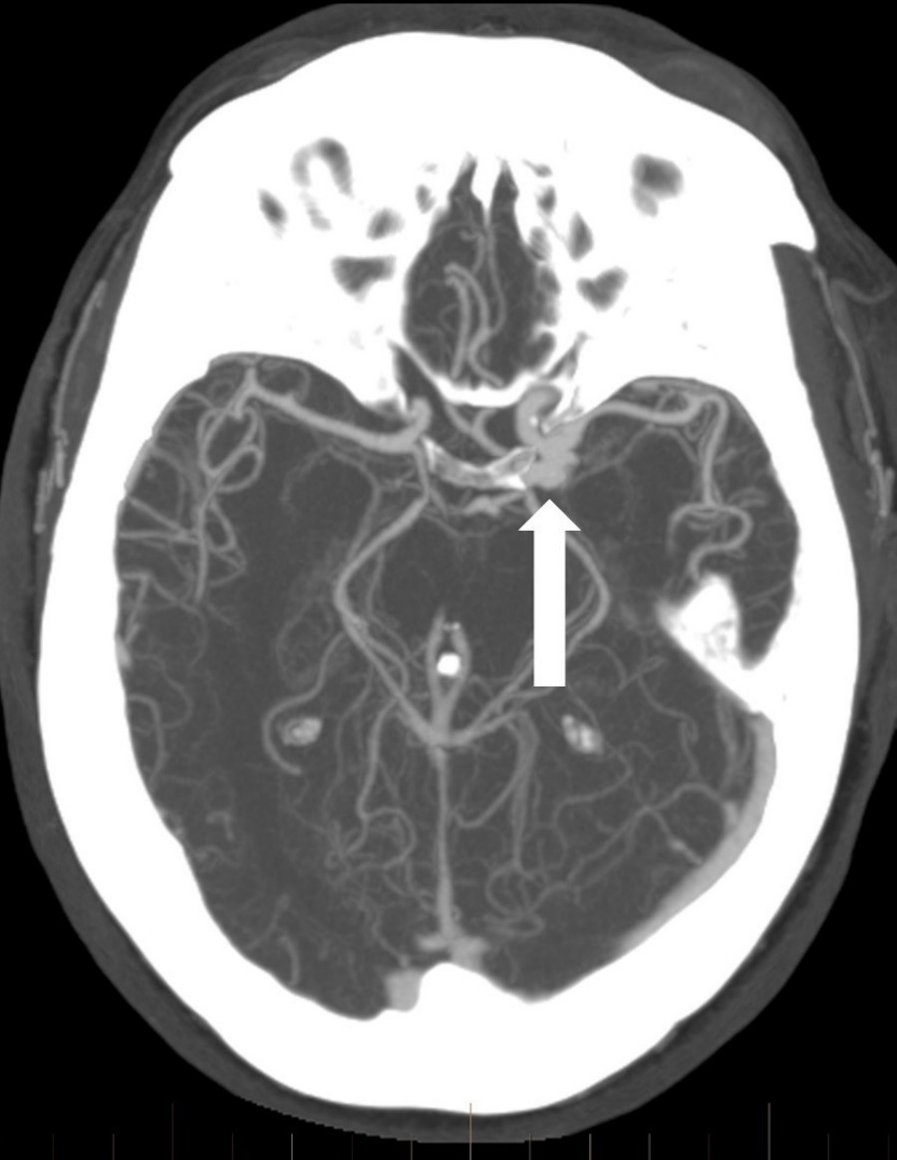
Axial computed tomography angiogram shows the wide neck multilobulated left posterior communicating artery region aneurysm (arrow).
